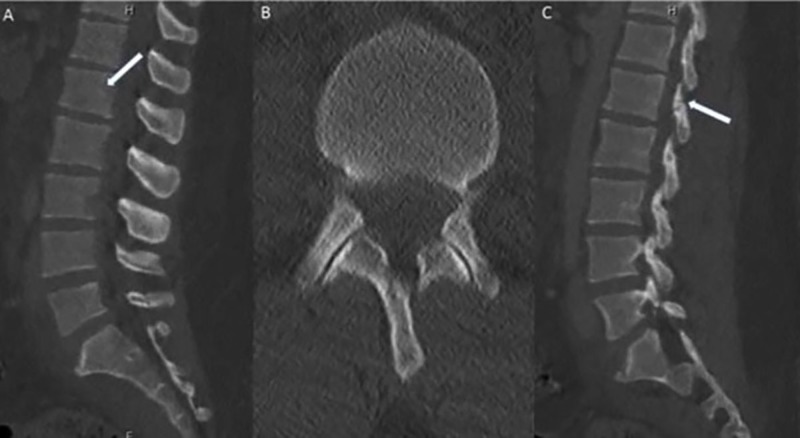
Admission CT scan (A) There is normal vertebral body alignment with a subtle fracture through the superior endplate of L1 extending to the posterior aspect of the vertebral body (white arrow). (B) Axial image of the L1-2 facet joints that show no sign of widening or abnormality. (C) Sagittal image showing linear hypodensity within the pars interarticularis of L1 concerning for acute fracture that was not seen on other reconstructions (white arrow). This was not reported on the original CT read. CT: computed tomography
